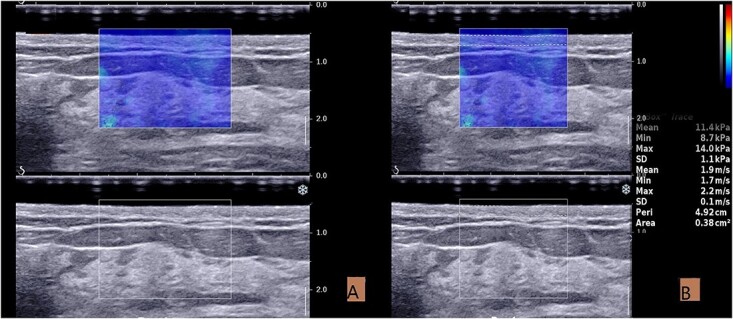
Acquisition and measurement of ultrasound images. (A) The SWE image taken in the resting state. (B) Measurement of elastic modulus in SWE mode. The skin boundary is traced manually to measure the elastic value of the entire skin layer.
